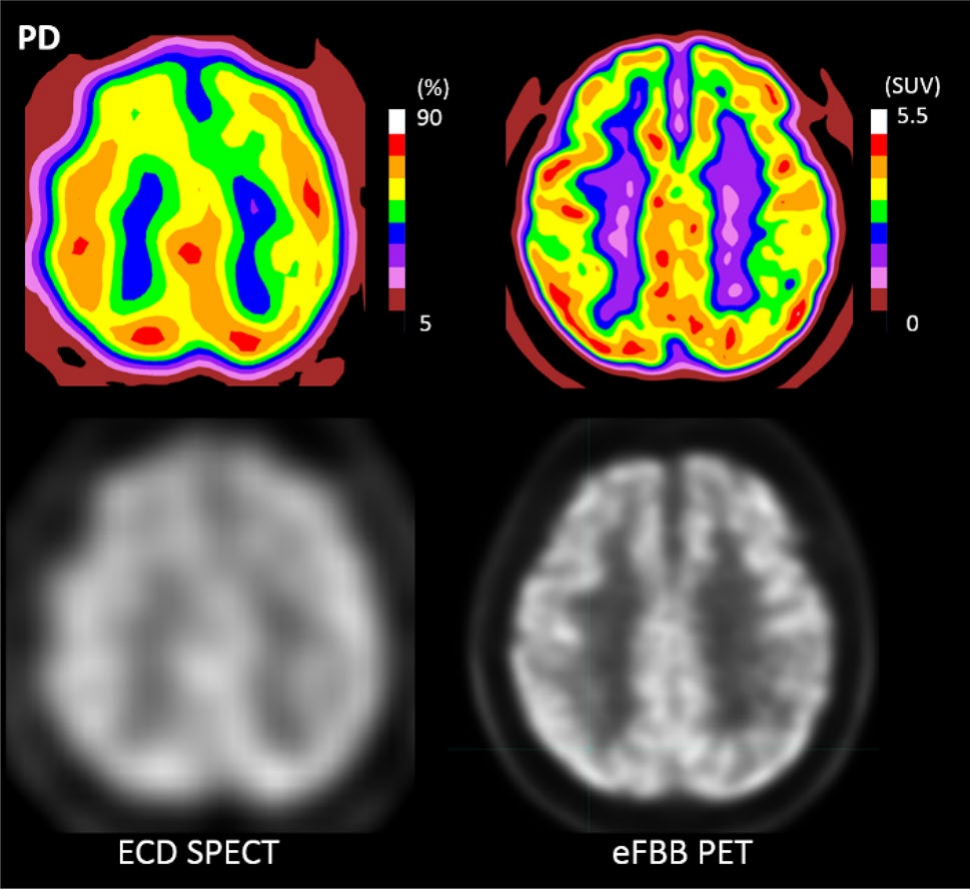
Sample images of ECD SPECT and eFBB PET scans of patients with PD. Sample images are visualized in 10-step colored images (upper row) and grey-scale images (lower row). Abbreviations ECD, Tc-99m ethyl cysteinate dimer; eFBB, early-phase F-18 Florbetaben; PD, Parkinson’s disease; PET, positron emission tomography; SPECT, single photon emission computed tomography; SUV, standardized uptake value.
